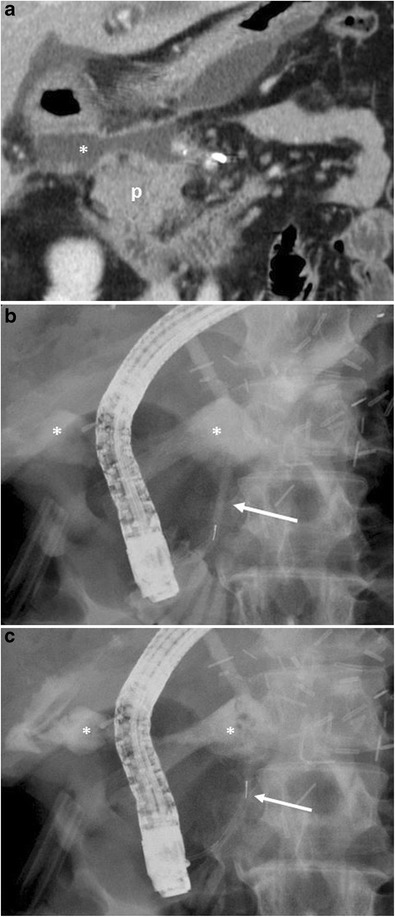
Pancreatic fistula. a Coronal curvilinear CT reconstruction shows the presence of a fluid collection (*) close to the resection margin of the pancreas (p) after a distal pancreatectomy. The presence of amylase from a surgical drainage (not shown in a) was consistent with a pancreatic fistula. b, c Spot images during ERCP show the passage of contrast material through the main pancreatic duct (white arrows) in the collection (*), a finding diagnostic for a leakage of pancreatic juice at the resection margin
